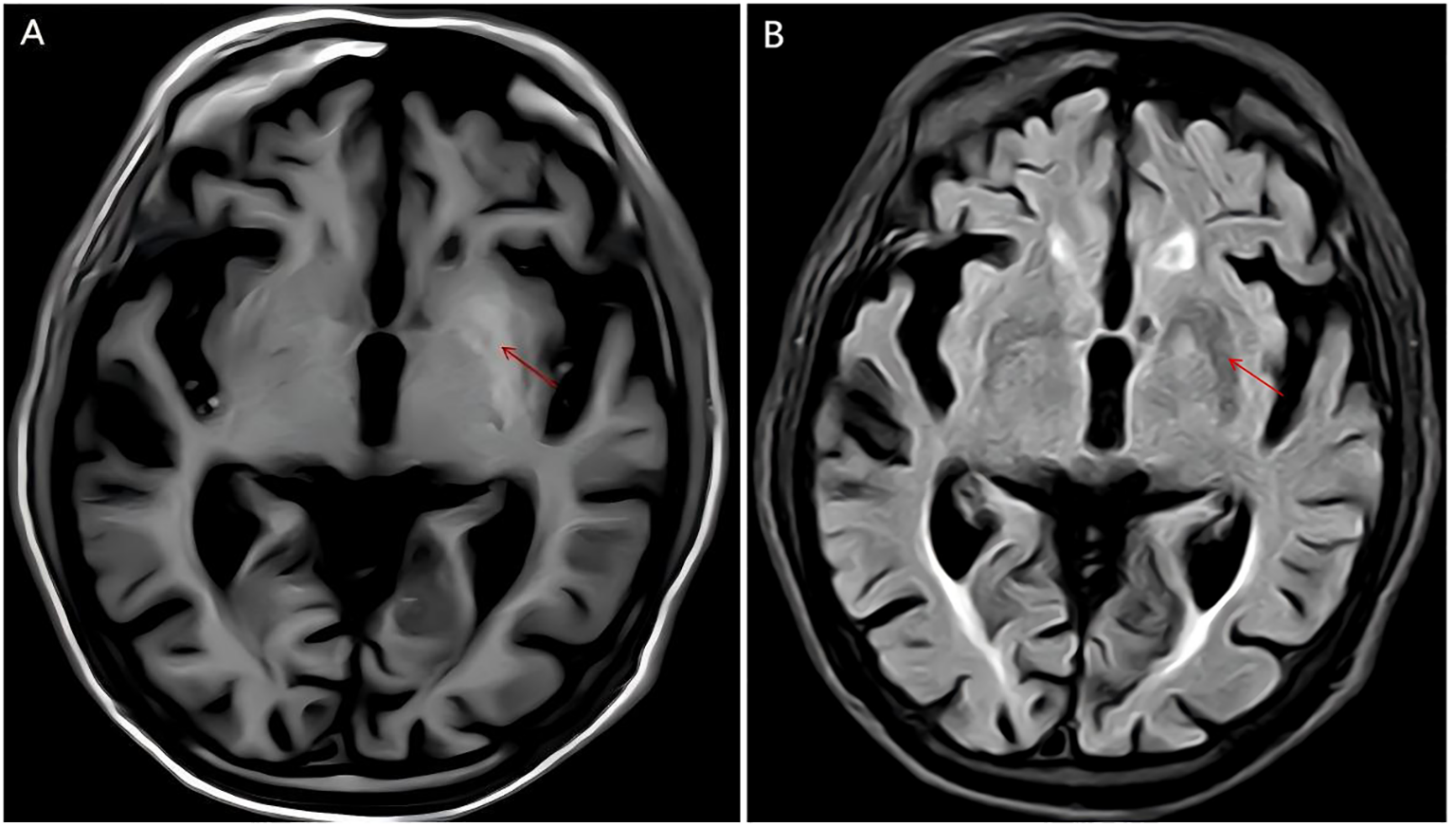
The MRI scan reveals patchy slightly shortened T1 signal foci in the bilateral basal ganglia regions, with ill-defined borders (A). T2-weighted imaging (T2WI) and fluid-attenuated inversion recovery (FLAIR) sequences show low signals, more pronounced on the left side (B). Abnormal signals were marked by red arrow.
